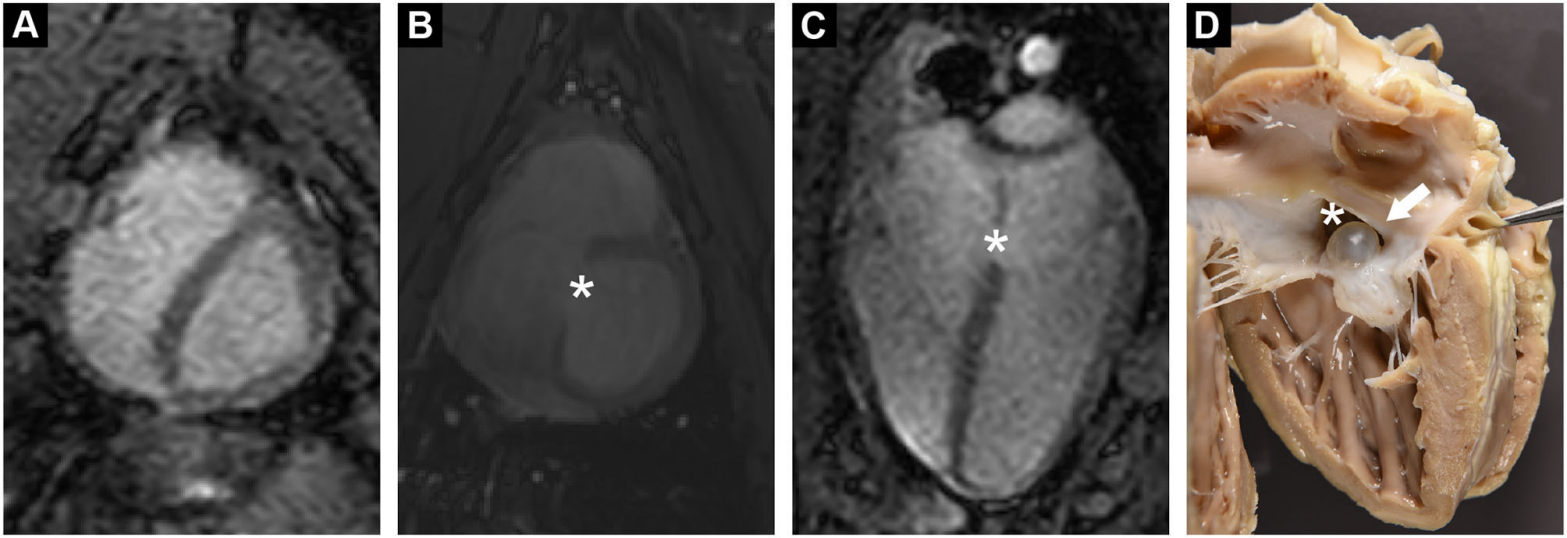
(A) Functional cardiac MRI in short axis revealed a diastolic D-shaped left ventricle, indicating increased right ventricular pressure. (B,C) Cardiac MRI in short and long axis demonstrated a tissue defect in the inter-atrial septum (*). (D) Post mortem examination confirmed a large ASD (*). ASD was partially occluded by a serous cyst (arrow).
